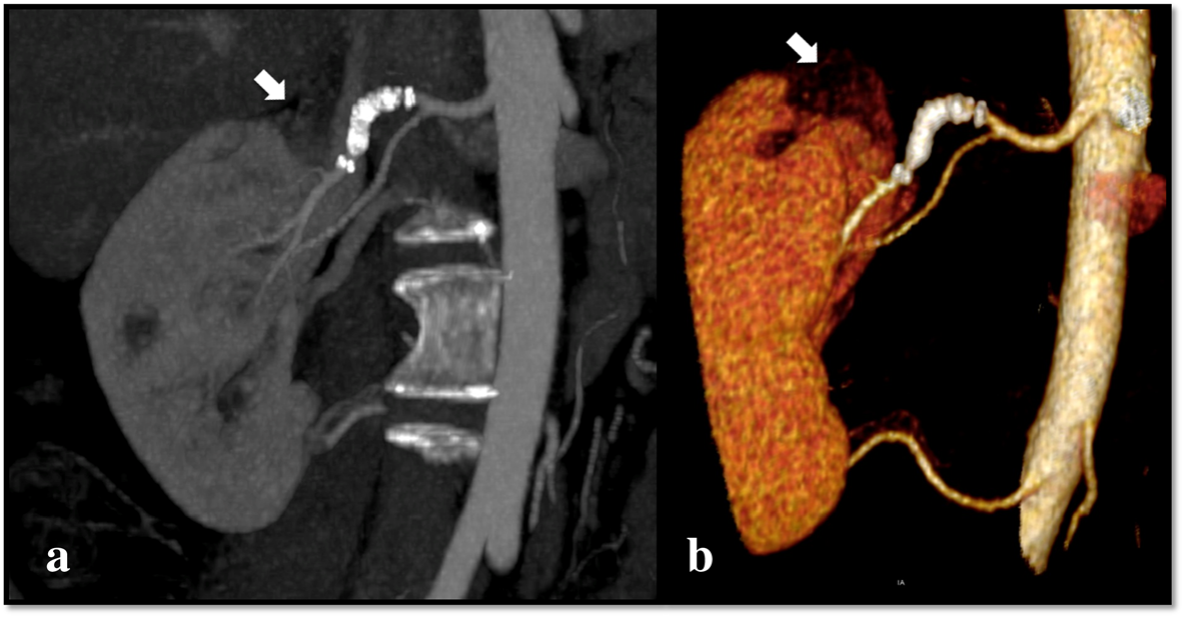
a, b Coronal CTA (a) and 3D volume rendered (VR) imaging (b) at 12 months demonstrating developing of complete exclusion of the aneurysm and the patency of the stent. A small area of hypoperfusion in the upper pole of the kidney is visible (large arrow)
